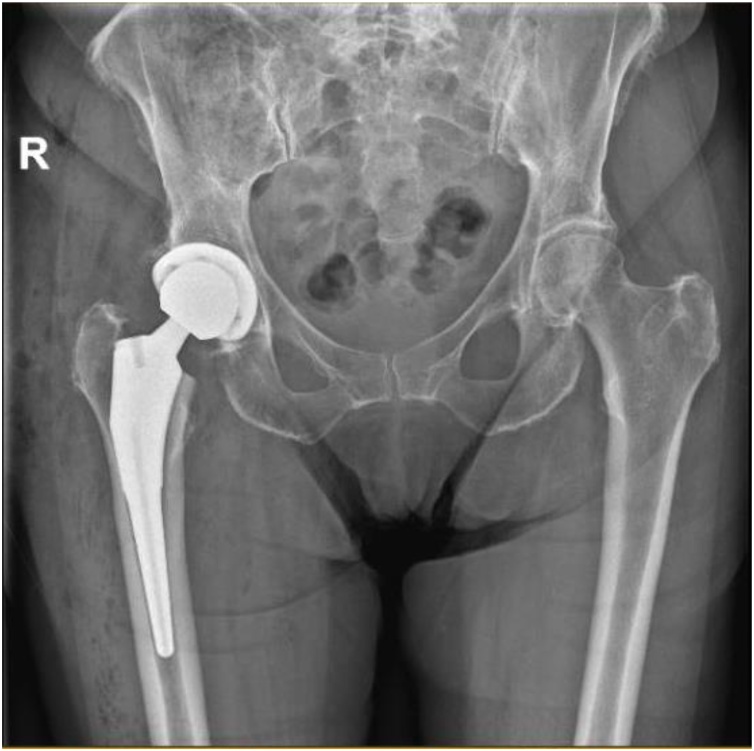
Plain X-ray shows no arguments of prosthetic loosening.
